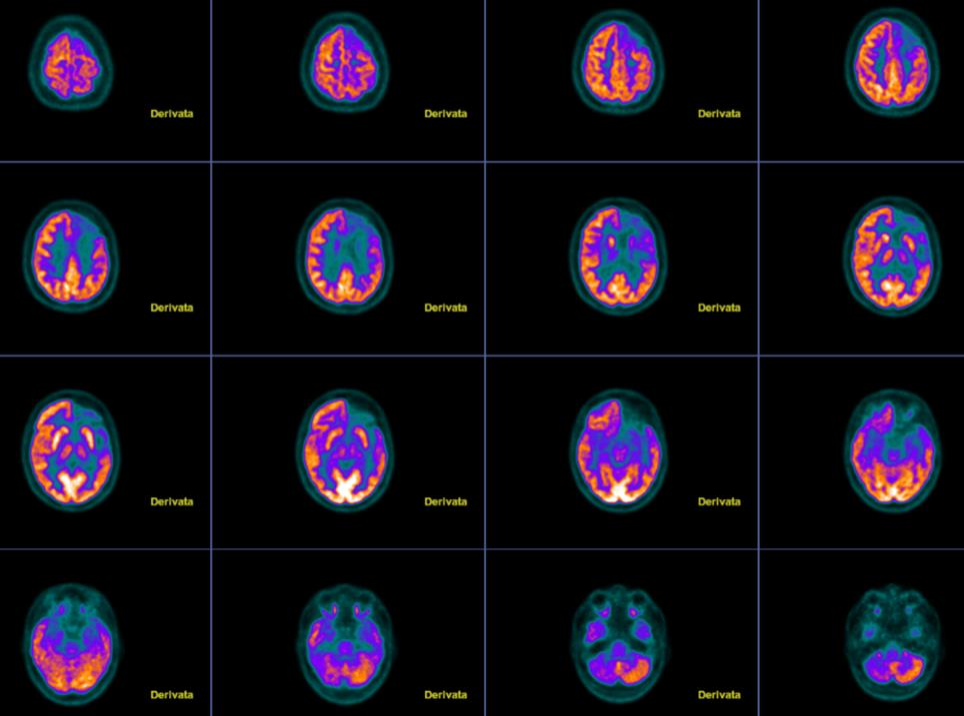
FDG-PET images with transaxial image: from the left upper corner in the upper row a diffuse unilateral hypometabolism in the left frontal lobe is showed. This pattern becomes more evident in the second row where the hypometabolism in the insular lobe and moderate hypometabolism in the left temporal lobe can be seen. At the bottom right corner, crossed cerebellar diaschisis is evident
